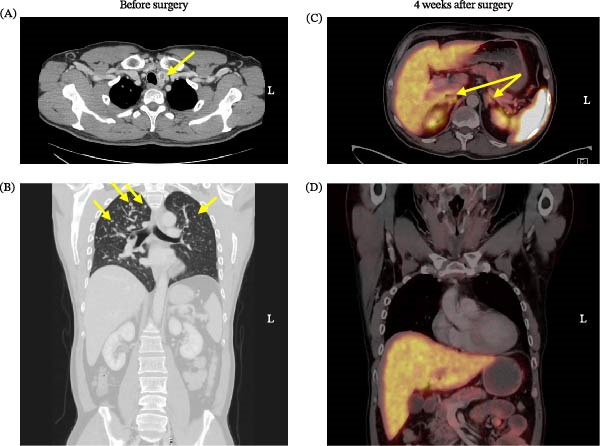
Selected radiological examinations of Case 1. CT images (A,B) prior to surgery reveal a tumor mass in the left thyroid lobe and multiple lung metastases. Yellow arrows indicate the left‐sided thyroid tumors in panel A and selected lung metastases in panel B. Approximately 4 weeks after thyroidectomy (C,D), a ^68^Ga‐DOTATOC‐PET/CT scan (C,D) was performed, showing no radiotracer‐positive tumors in the neck, lungs, or liver, but enlarged adrenals bilateral indicated with yellow arrow (C). Transverse (A and C) and coronal (B and D) sections are presented, and “L” indicated left side.
